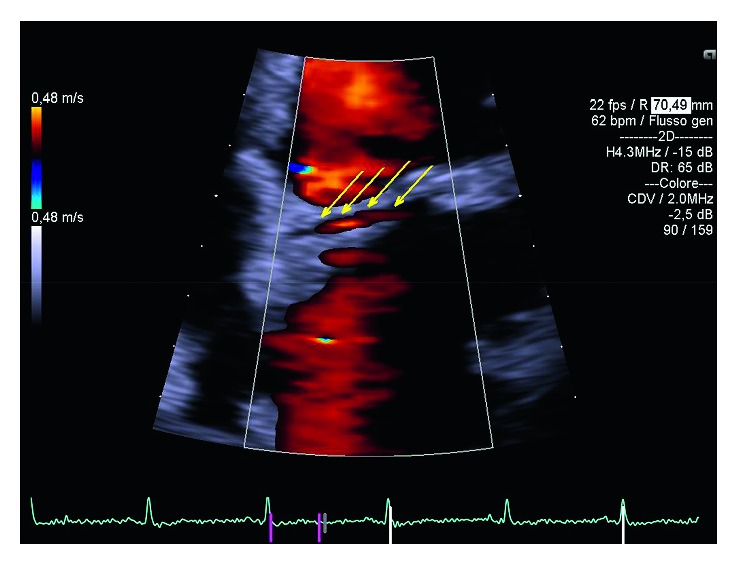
Presentation of anomalous circumflex course. Arrows and color-Doppler indicate its course.
